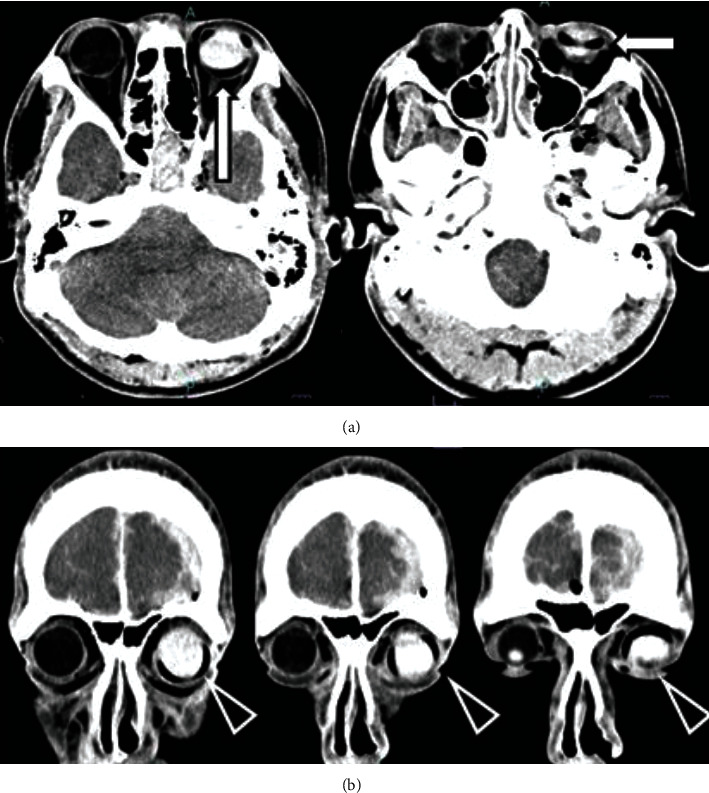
(a) Axial transverse CT and (b) coronal CT through the orbit. A low-density silicone rubber circling band (white arrow) and a well-defined, homogenous, hyperdense mass (black arrowhead) seen in the cross section were observed in the left eye.
